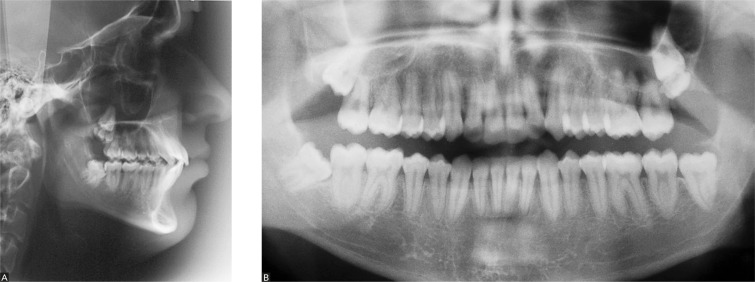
Post-treatment radiographs. A) Cephalometric radiograph; B) Panoramic
